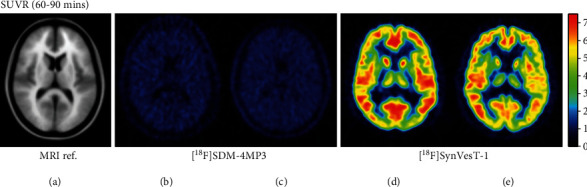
Average axial views in MNI space. (a) MRI shown for reference of SUVR, 60-90 min, centrum semiovale reference tissue, for subjects imaged with [^18^F]SDM-4MP3 (b, c), and [^18^F]SynVesT-1 (d, e).
